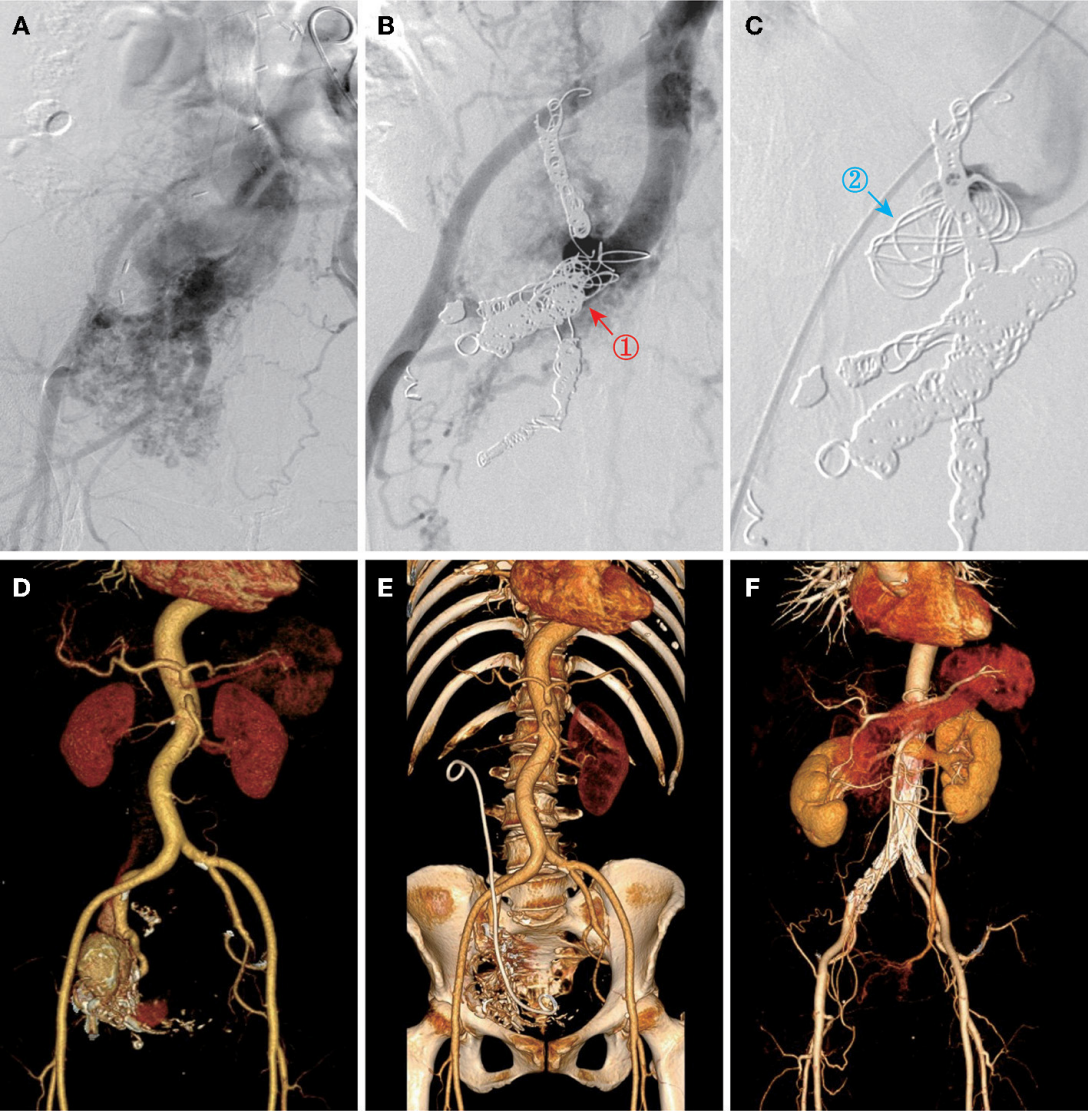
Other three types of endovascular approach. (A) Angiography showed IAVFs in a 49-year-old female (case 9). (B) Transarterial embolization of inflow right internal iliac arteries on stage I (red arrow and “”). (C) Transvenous embolization of outflow right internal iliac vein on stage II (blue arrow and “”). (D,E) Pre- and postoperative CTA images of a 56-year-old female (case 8) who was performed transarterial and transvenous embolization once time for right internal IAVFs. (F) Postoperative CTA image of a 53-year-old female (case 5) who was performed transarterial embolization plus stent placement for bilateral common IAVFs.
